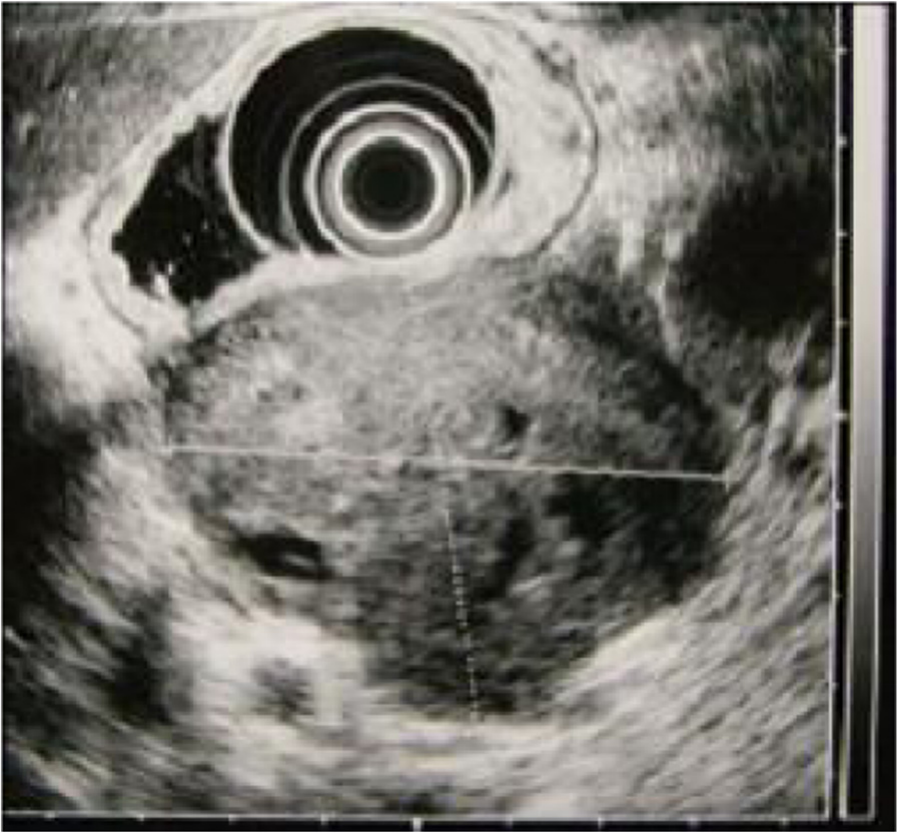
Endoscopic ultrasonographic findings of the tumor. Endoscopic ultrasonography demonstrated a heterogeneous lesion 60mm in diameter, arising in the posterior wall of the stomach.
